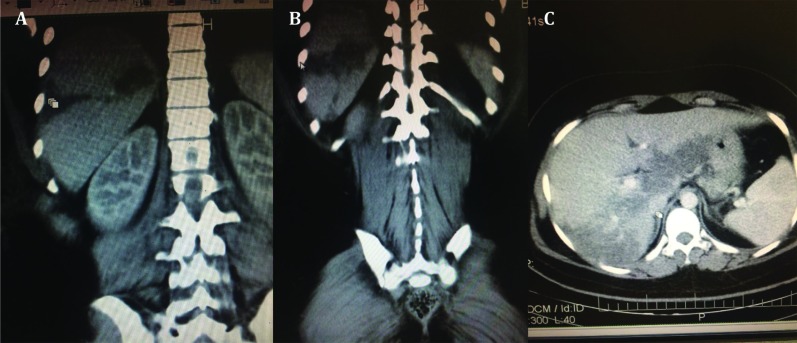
Abdominal CT-image. A & B: coronal view; C: Plain scan axial view of the lesion: demonstrating an intraparenchymal liver hematoma in the patient
